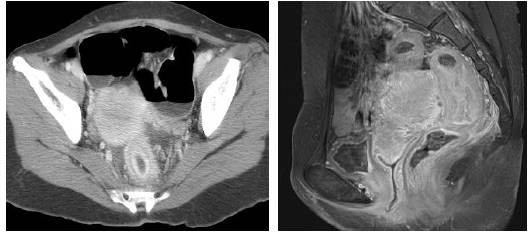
A. Axial abdominal CT demonstrates concentric wall thickening, particularly in the inner enhancing layer with a target pattern. B. MRI scan shows no evidence of tumor infiltration around the perirectal fat plane.
